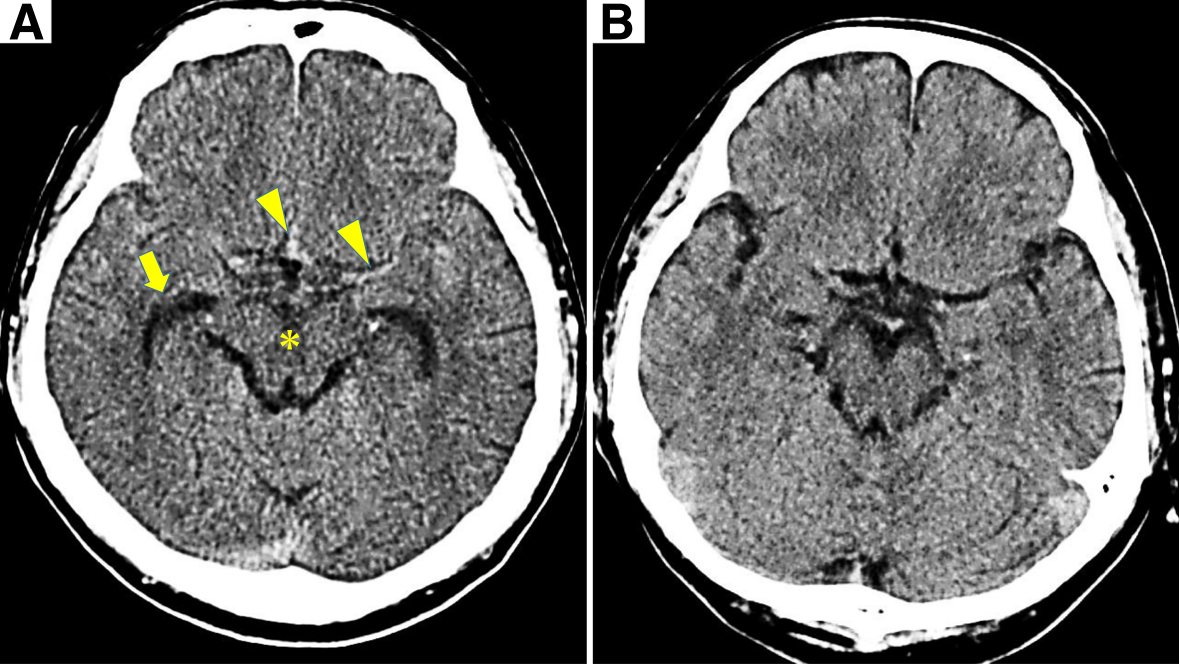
The slice is at level of the midbrain (mesencephalon) that traverses the infundibulum of third ventricle, interpeduncular cistern. In a, there appears to be hyperattenuating blood in the medial portion of the left Sylvian fissure and the anterior interhemispheric fissure, and shows mild dilation of the temporal horns of the lateral ventricles (looks like a moustache). In the center of the suprasellar cistern is CSF (hypoattenuating) in the dilated infundibulum of the third ventricle. These CT findings become clear in comparison to the previous CT (b). The SAH seen is in proximity to this left ACA aneurysm. An *arrow* indicates the temporal horns of the lateral ventricles. Arrowheads indicate hyperattenuating blood. An *asterisk* indicates CSF (hypoattenuating) in the dilated infundibulum of the third ventricle
